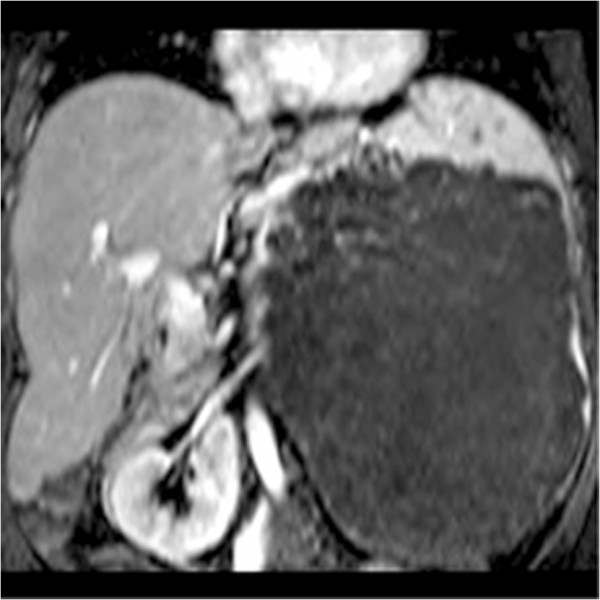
Coronal T1-weighted plus gadolinium magnetic resonance imaging of splenic hamartoma.
